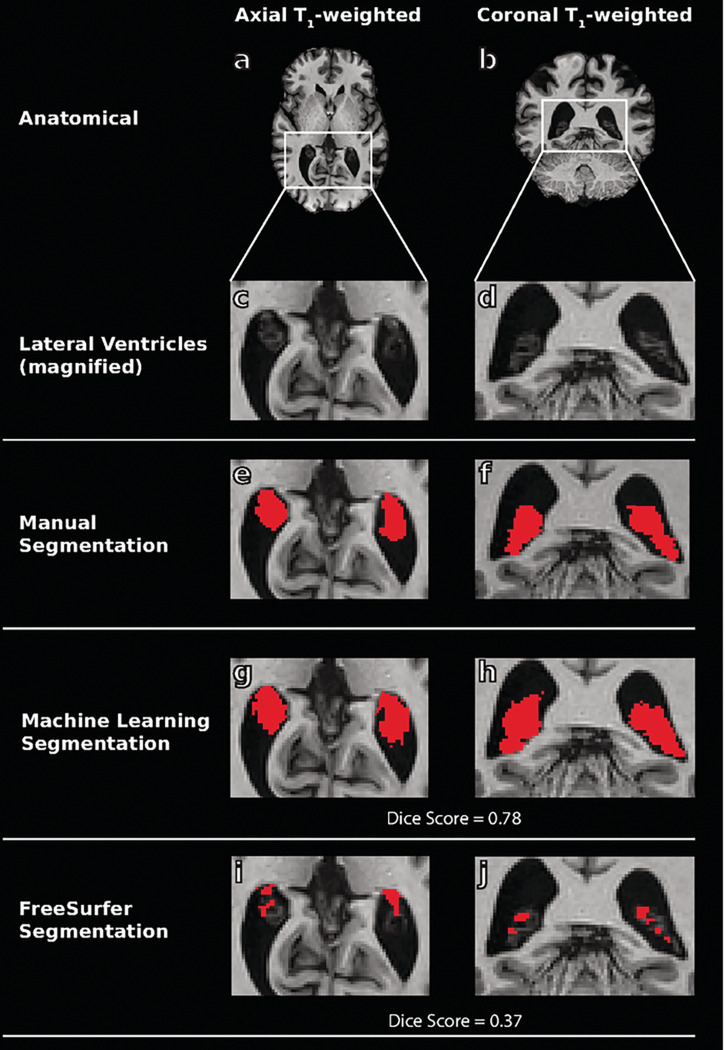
Example T_1_-weighted magnetic resonance images (MRI) from a 53-year-old male with mild cognitive impairment (a-d) and manual tracings (e-f) utilized in training of the machine learning methods. Example outputs from the T_1_-weighted-trained machine learning model (g-h) are shown compared to FreeSurfer segmentations (i-j). Dice scores are shown for machine learning and FreeSurfer outputs and reflect an improvement in segmentation accuracy for the proposed method.
